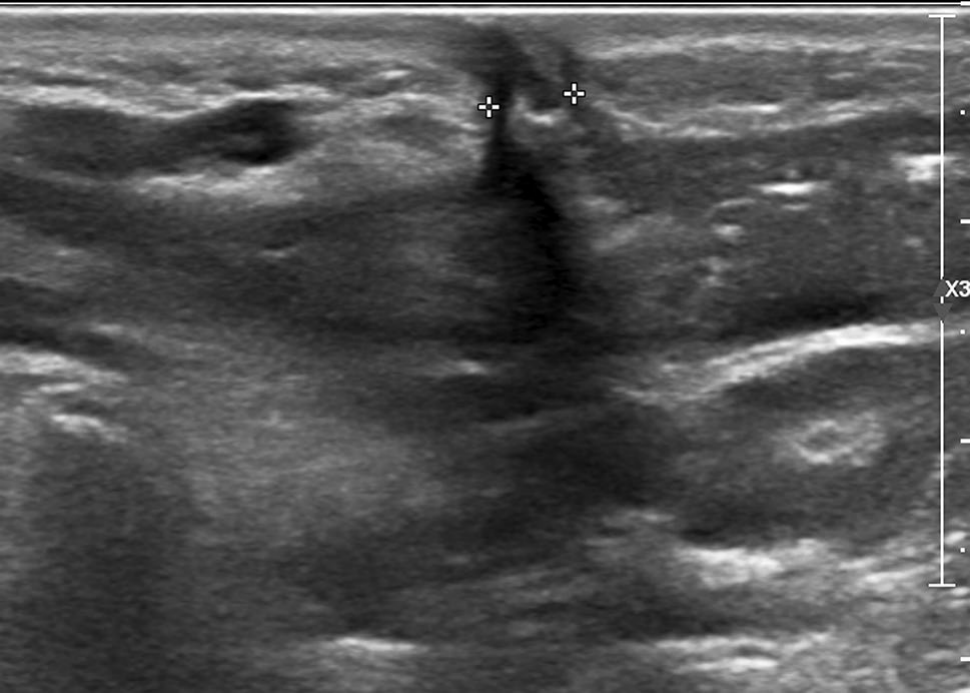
Cervical ultrasonography of lymph nodes shows abscess and sinus. Subcutaneous abscess is formed near the lymph nodes with ill-defined boundaries, and strips of hypoechoic sinuses are seen above extending into the skin
